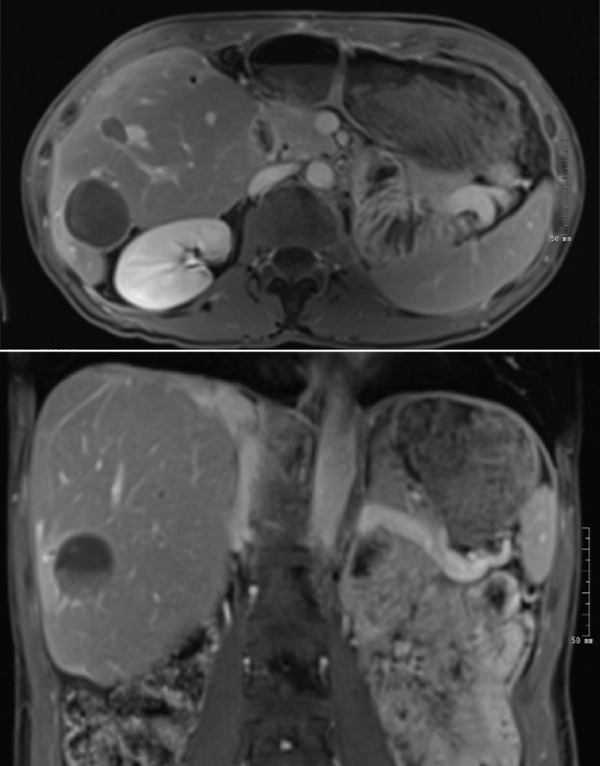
MRI abdomen with multiple foci of artifact throughout the liver consistent with ablation posttreatment changes, and interval decreased size in hepatic foci suggesting treatment response. There is no evidence of disease progression.
